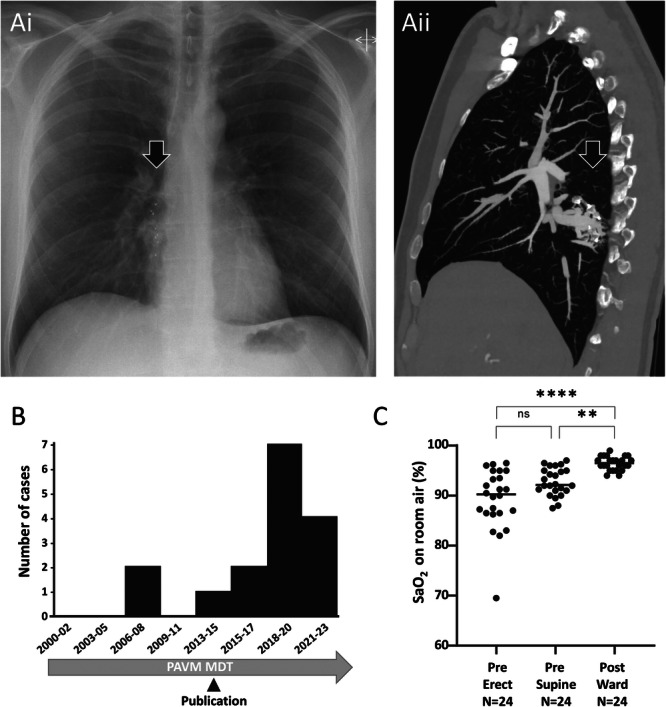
(A) Complex right lower lobe PAVM following previous embolization with several Amplatzer vascular plugs. Preoperative images. (i) Chest x‐ray indicating Amplatzer plug radiopaque tips and additional soft tissue shadowing (arrowed). (ii) Sagittal images from a contrast enhanced thoracic CT scan demonstrating a persistent venous sac with multiple small residual feeding arteries, the diameter and position of which preclude complete embolization. (B) Case series: Serial number of elective thoracic surgical cases, noting date overseas audit of cumulative radiation doses in patients with PAVMs was published [4]. Cases from the third series (2023‐) are the subject of separate Case Reports with specific educational/training foci. (C) Pre and postoperative oxygen saturation (SaO_2_). SaO_2_ is a validated biomarker of right‐to‐left shunting [5], and did not differ between Series 1 and Series 2 pretreatment (means 89.2% *vs.* 89.6%). *p* values were calculated by Dunn's test post Friedman (*p* < 0.01, *p* < 0.001).
